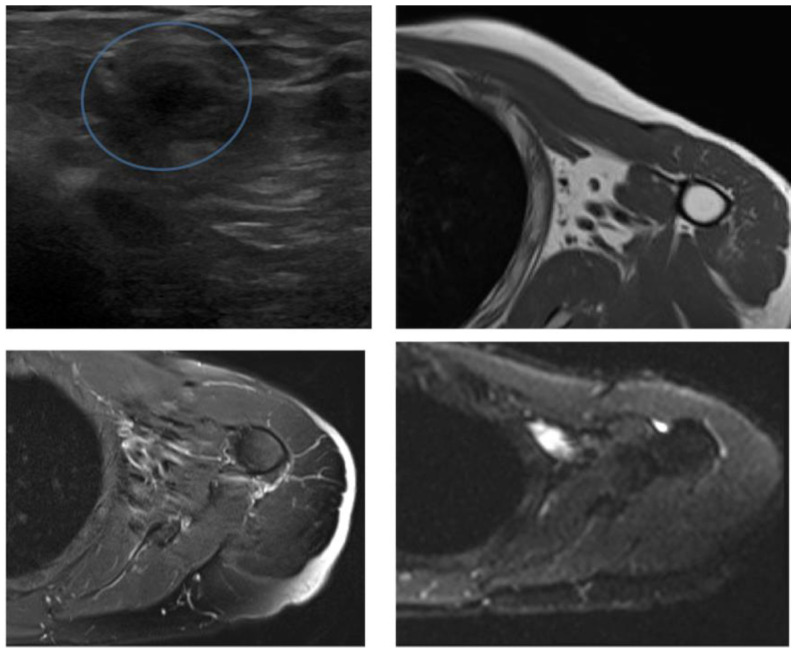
A 71-year-old man with intermediate-grade leiomyosarcoma of the proximal arm. US was reported as positive for recurrence due to the detection of a pseudomass/irregularity of the superficial tissues of the proximal arm (circle). MRI did not show any mass. Follow-up did not show any recurrence.
